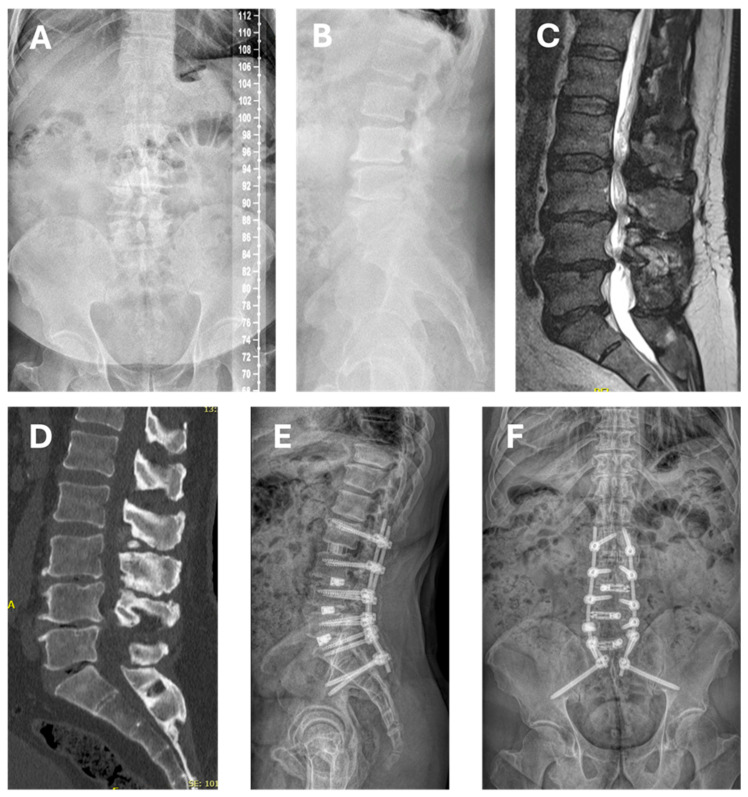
Case vignette of a 64-year-old male with history of three prior lumbar decompression procedures (hemilaminectomy L5, foraminotomy L5, bilateral decompression L3/4), presenting in our outpatient clinic with neurogenic claudication, recurring spinal stenosis, severe foraminal stenosis at multiple levels, and motor deficit for elevation of the left foot (BMRC grade 2/5). He received multiple epidural steroid injections and facet blocks with only temporary improvement of symptoms. (A,B) Standing preoperative whole-spine X-ray in anterior–posterior and lateral projection (PI 29°, Roussouly type 1, PT 5°, SS 24°, LL L1-S1 46° (ideal: 43° according to www.spinebit.io, accessed on: 27 July 2025), SL L4/S1 28° (ideal: 39° according to www.spinebit.io)). (C) Sagittal MRI demonstrating spinal stenosis at L2/3, L3/4, and L4/5. (D) Sagittal CT scan with evidence of degenerative disc disease and vacuum sign at L3/4, L4/5, and L5/S1. Considering the severity of his symptoms (VAS back pain 7/10, VAS leg pain 10/10, Oswestry Disability Index (ODI) 53.3/100%, Core Outcome Measures Index (COMI) Back 8.14/10), a fusion procedure was proposed entailing indirect decompression at L2/3 via extreme lateral lumbar interbody fusion (XLIF), as well as direct decompression with TLIF at L3-S1. (E,F) Postoperative standing whole-spine X-ray in anterior–posterior and lateral projection demonstrates good restoration of SL between L4-S1 (37°) and between L1-S1 (42°). The patient recovered well, without suffering from intra- or postoperative complications. At 12 months, he presented with significant improvement of preoperative symptoms (VAS back pain 3/10, VAS leg pain 1/10, ODI 22/100%, COMI Back 2.28/10) and without signs of pseudarthrosis.
